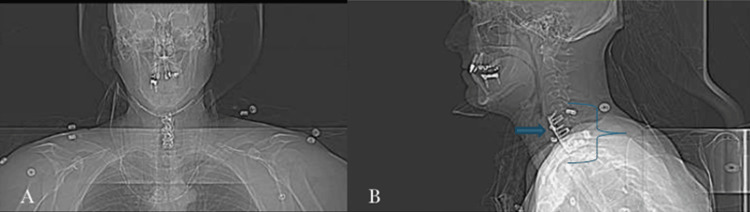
Non-contrast CT scan of the spine showing (A) anterior-posterior and (B) lateral views of the patient with anterior fusion plates spanning C5-T1 (blue arrow) and microscrews anchored within the C5, C6, and T1 vertebral bodies (blue bracket). Intervertebral disc spacers are also seen without osseous fusion across the intervertebral disc spaces.
